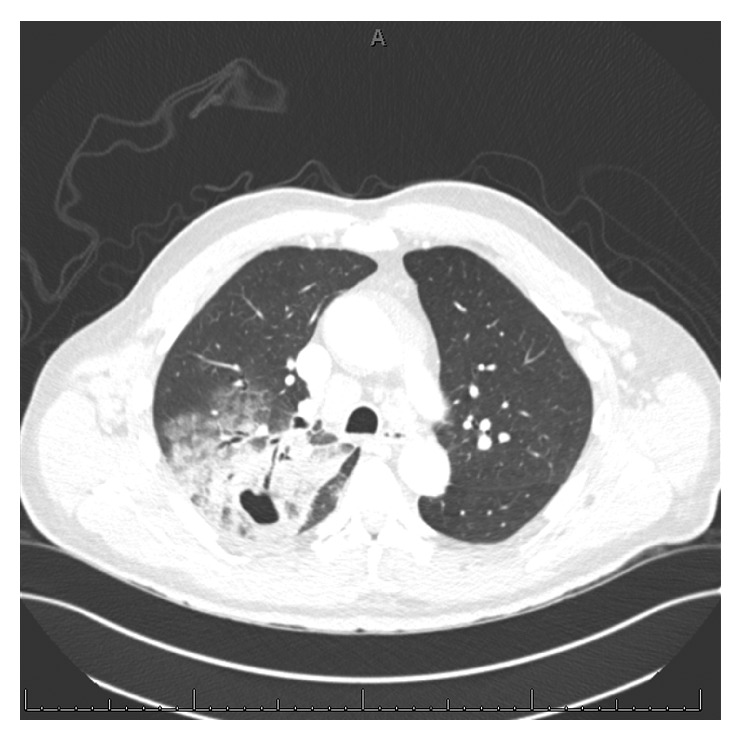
Contrast-enhanced computed tomography of the chest showing a cavitary mass with associated ground glass opacities and pneumonia.
